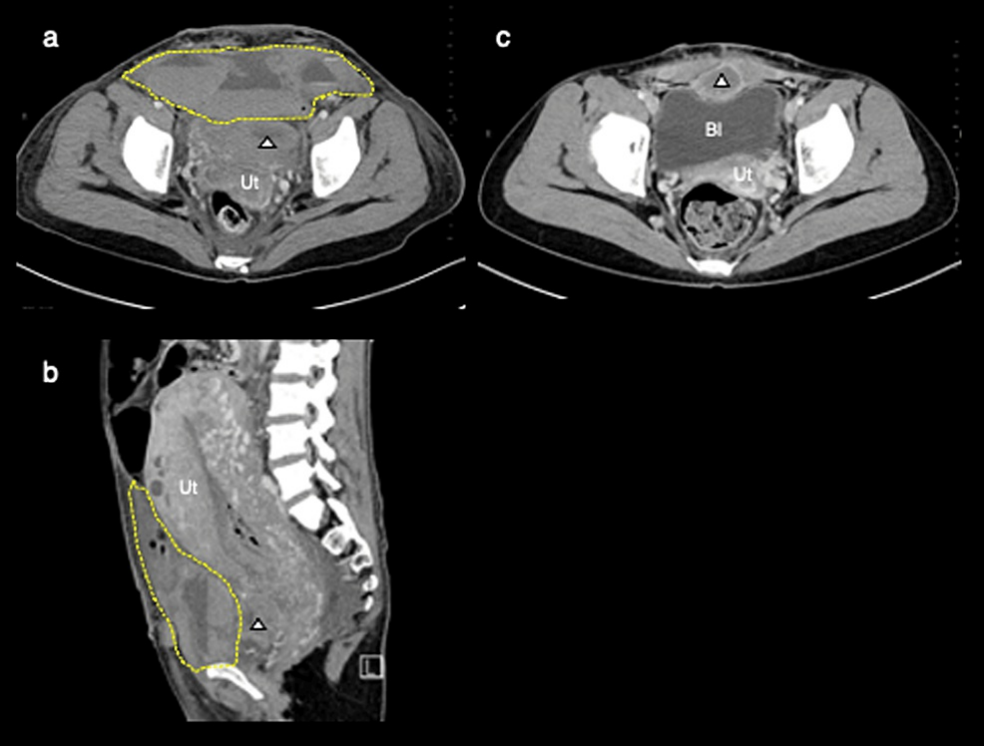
Imaging of rectus sheath hematoma on CT. (a,b) The area surrounded by a yellow dotted line shows the hematoma, including the rectus abdominis muscle, of size 16×5×8 cm on POD 2. The white triangle shows a hematoma at the vesicouterine pouch. (c) On POD 92, the rectus sheath hematoma already disappeared. White triangle shows hematoma at the vesicouterine pouch. Ut, uterus; Bl, bladder; CT, computed tomography; POD, postoperative day
